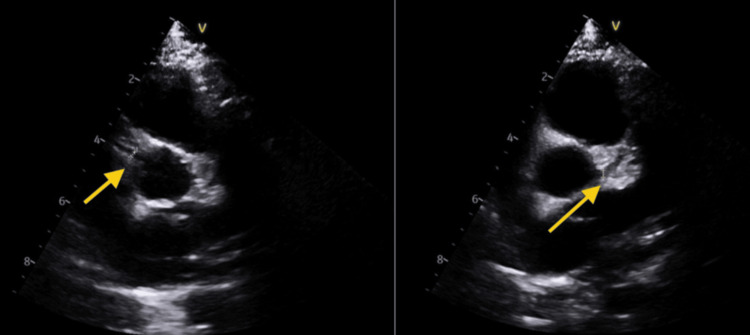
Echocardiography at the two-week follow-up Left arrow: RCA 2 mm; Right arrow: LMCA 2.2 mm RCA: right coronary artery; LMCA: left main coronary artery
